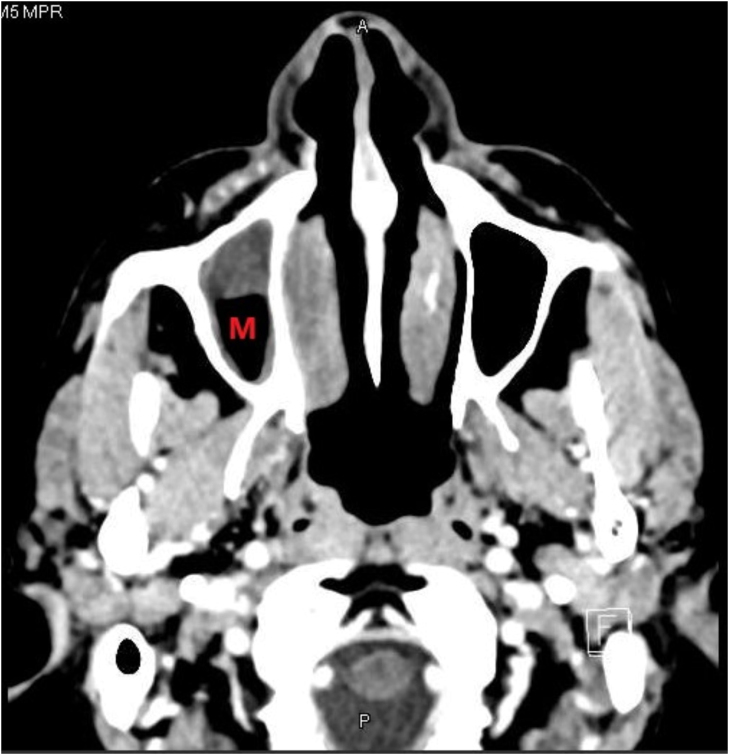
C.T. Head with contrast showing right maxillary sinus opacification (red "M" letter). CT: Computed Tomography.
